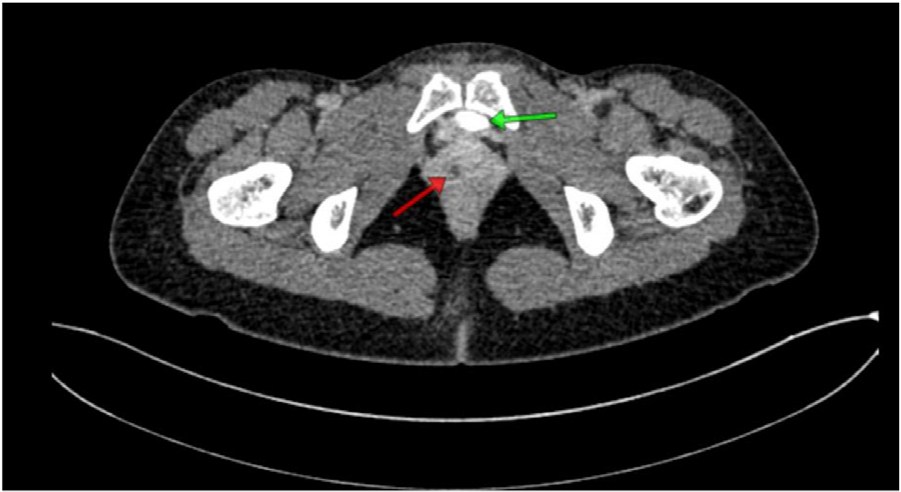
Contrast-enhanced abdominal CT scan: The urinary bladder (green arrow) and the distal right ureter are seen opening into the vagina (red arrow).
